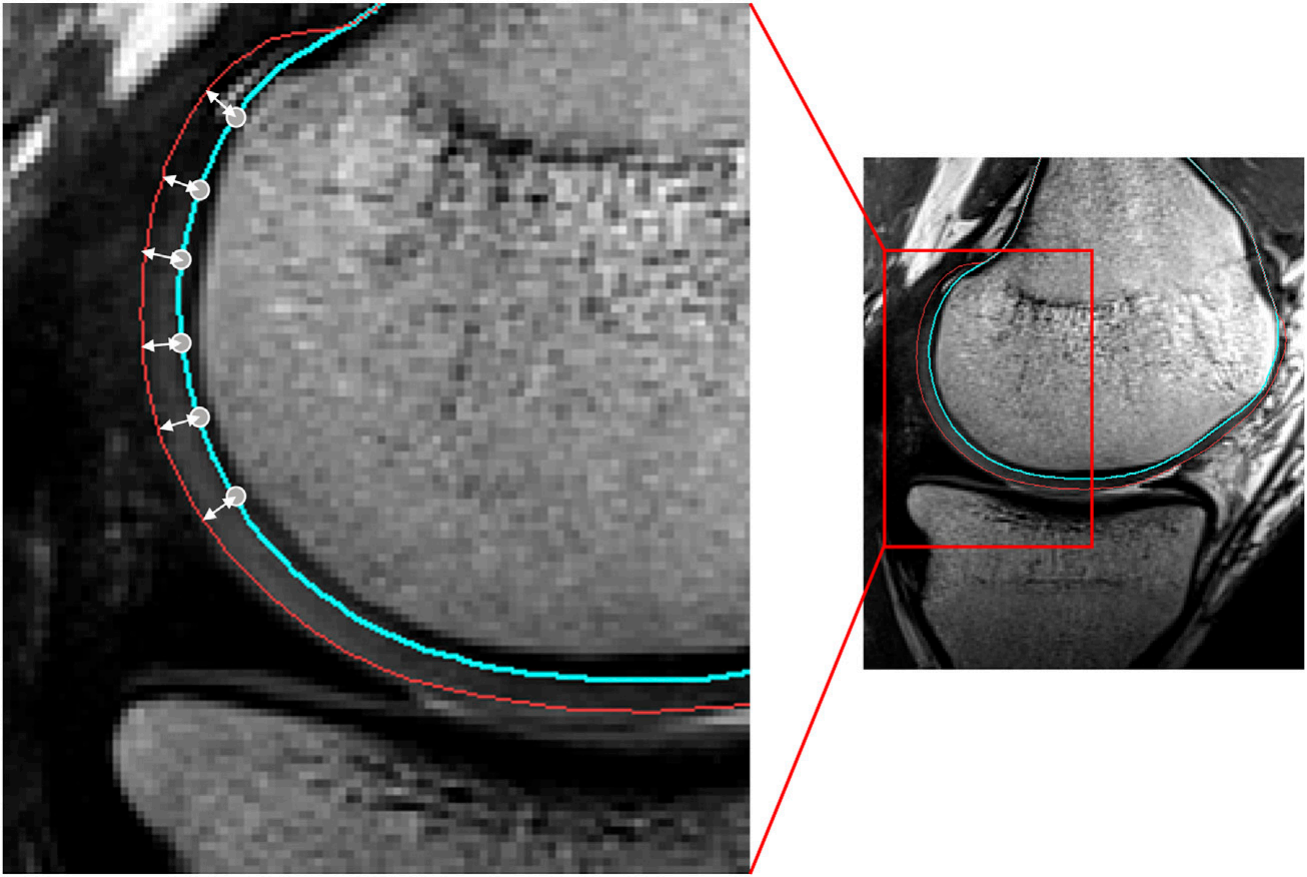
Sagittal view of the femoral condyle on MRI. The node-specific cartilage thickness was defined as the distance of the vertex (located on the blue line) to the cartilage surface (red), along the vertex normal.
